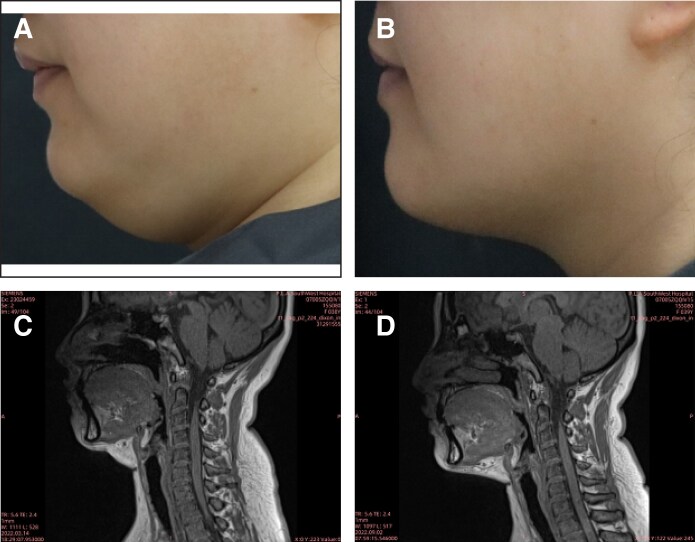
A 38-year-old female treated with MEI005. (A) and (B) reveal pretreatment and improved jawline contour, respectively, at 12 weeks after the fourth treatment session (Week 12), whereas (C) and (D) reveal reduced submental fat volume on MRI at the same time points.
